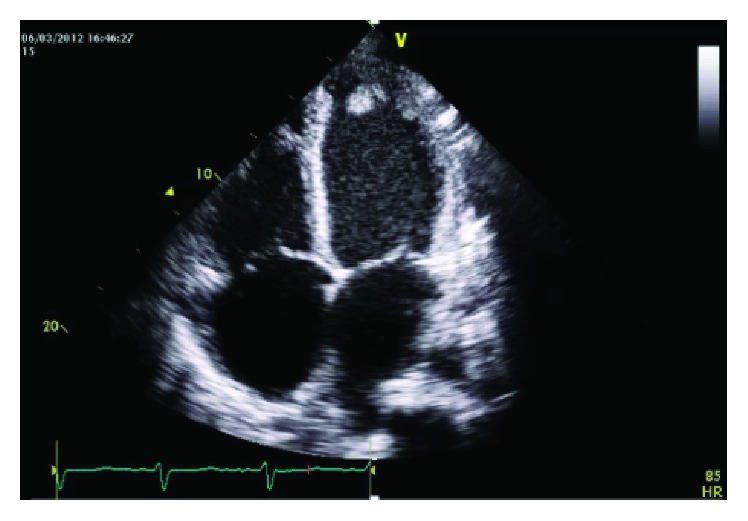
Transthoracic Echocardiogram (TTE). A left ventricular apical intramural thrombus can be visualized.
